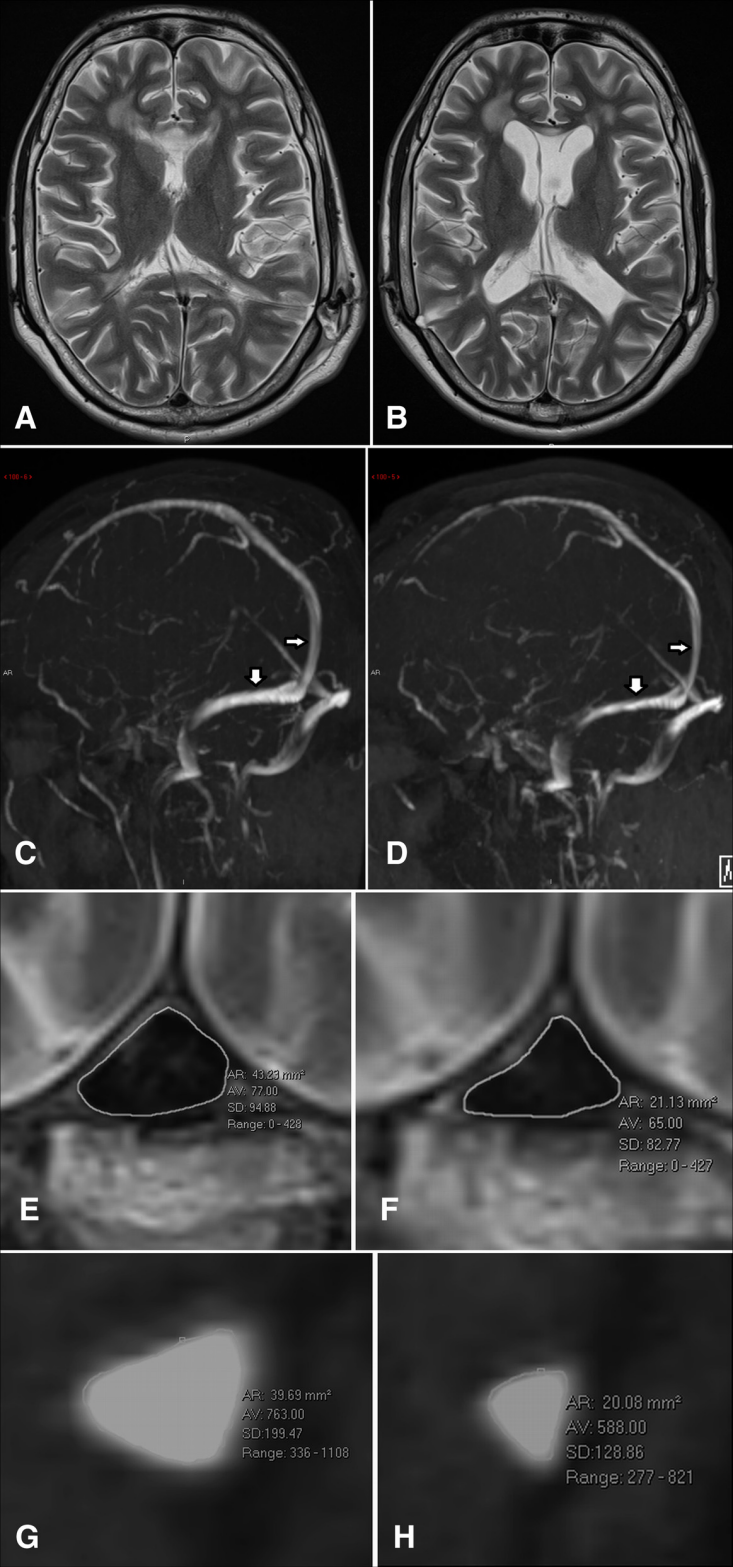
MRI imaging of a chronic hydrocephalus patient before and after shunt failure. A A T2 axial image of a 32 year-old male with chronic hydrocephalus and a left occipital shunt currently functioning. B A T2 axial image following removal of the shunt showing enlargement of the ventricles. C The MR venogram with functioning shunt appears normal, the thin arrow indicates the sagittal sinus and the thick the dominant transverse sinus. D The MR venogram with the shunt removed shows the sagittal and transverse sinuses to be smaller than previously. This is most easily seen at the level of the arrows. E A T2 axial image of the sagittal sinus 2 cm above the torcular taken at the level of the thin arrows in C and D) showing the cross-sectional area of the lumen to be 43 mm^2^. F The follow-up T2 axial image at the same level as 1e but with shunt removed shows the lumen to be 21 mm^2^. G A sagittal reconstruction of the mid portion of the right transverse sinus (at approximately the level of the thick arrows above) taken from the MRV raw data shows the sinus to be 40 mm^2^ in area. H The same reconstruction as 1 g following shunt removal shows the sinus area to be 20 mm^2^.
